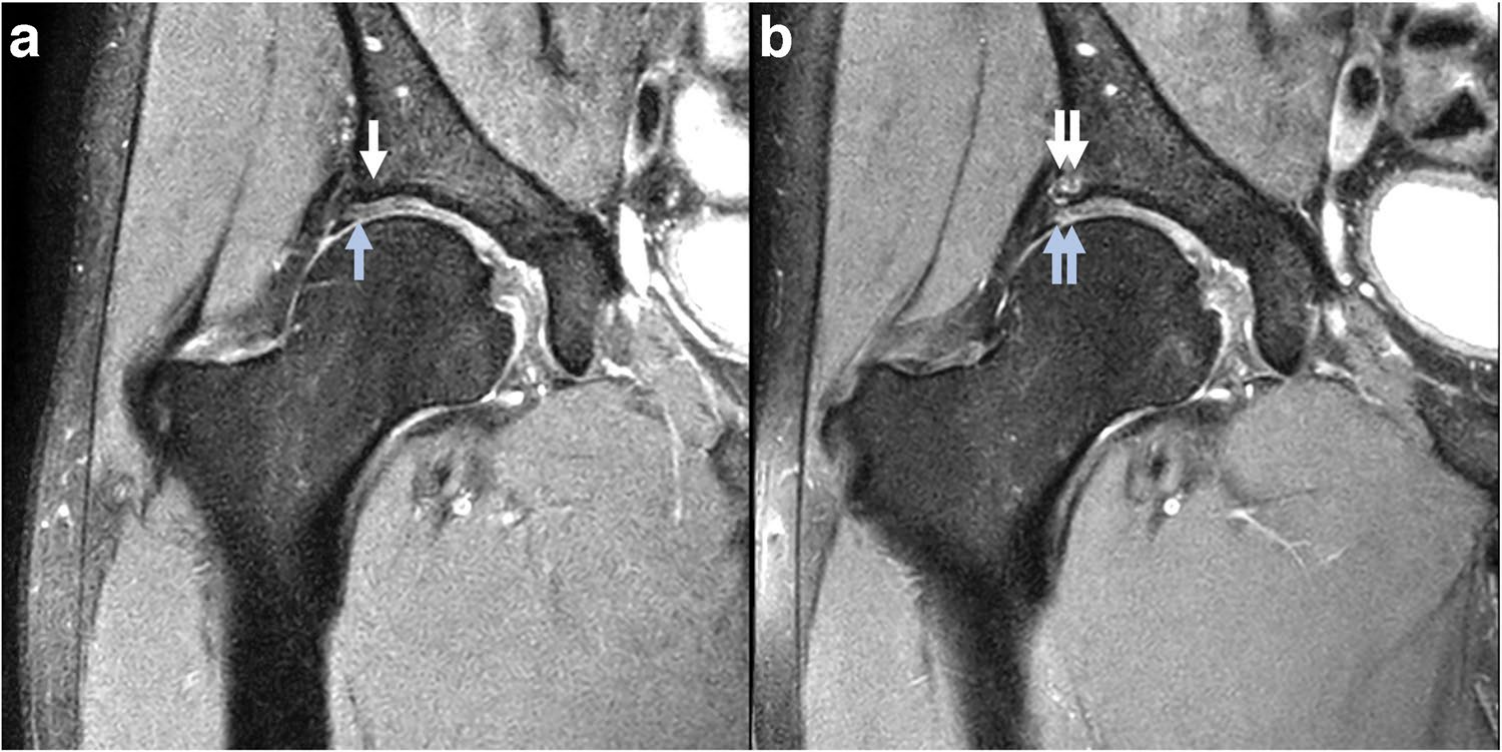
Incident cartilage defect and BML. a Normal subchondral bone (white arrow) and articular cartilage (blue arrow) in the superolateral subregion. b Two-year follow-up MRI shows incident grade 2 BML (double white arrow) and full-thickness (grade 2) acetabular cartilage defect (double blue arrow) in the superolateral subregion
